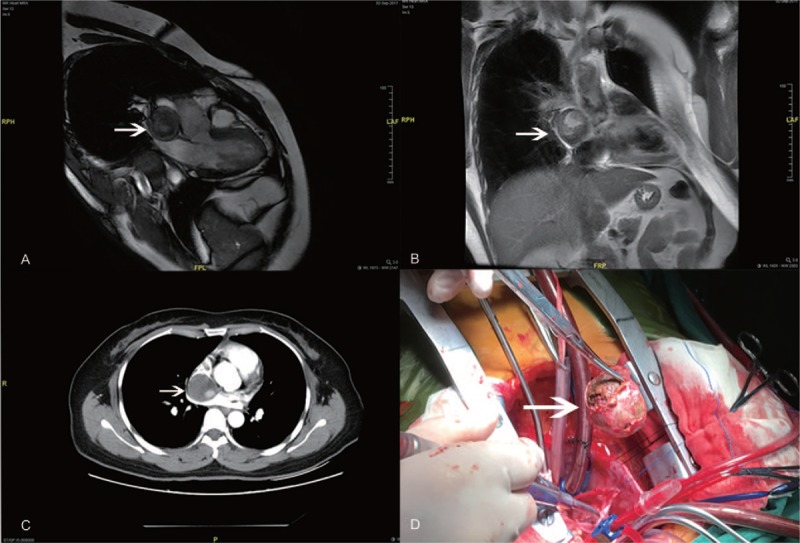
The magnetic resonance imaging and contrast enhanced computed tomography shows a heterogeneous mass without enhancement (A, B, and C). The mass was peeled thoroughly from the septum during operation (D).
